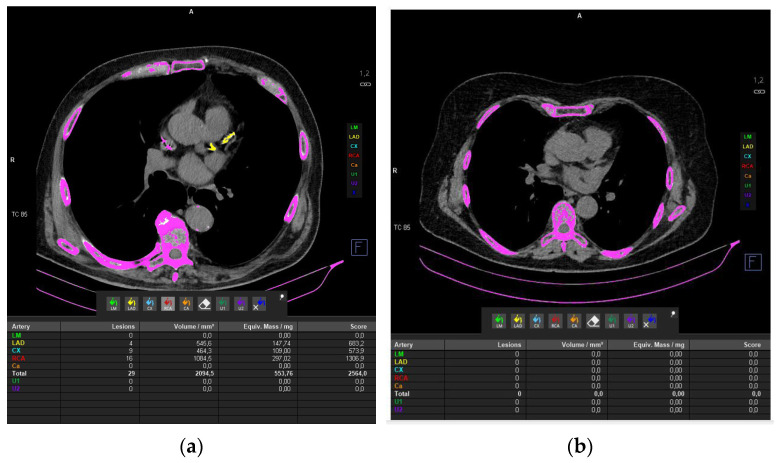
(a) shows CT images of the patient with the highest CAC score, whereas (b) shows CT images of a patient with a normal calcium score.
